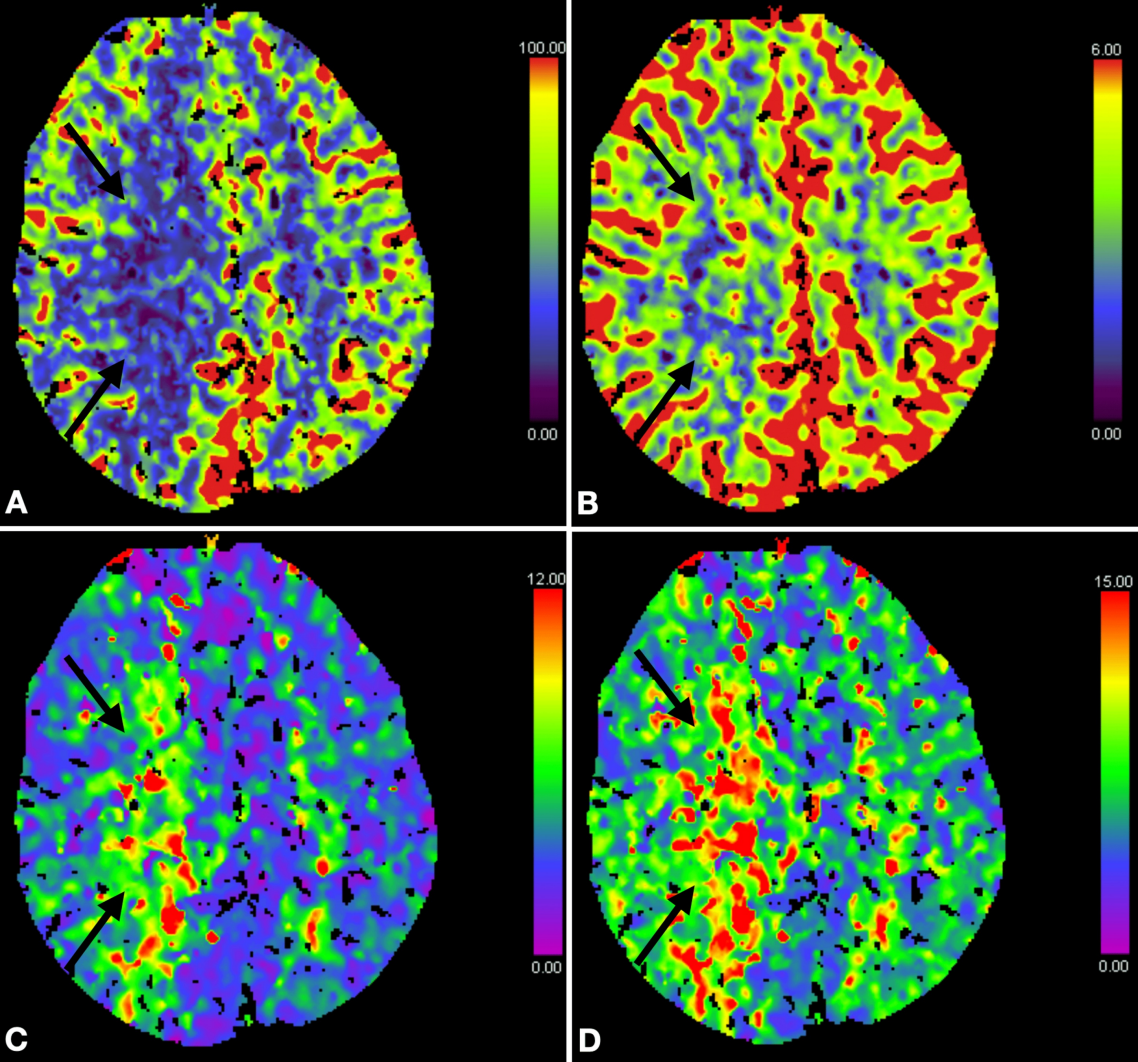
Computed tomography perfusion maps (A) Cerebral blood flow, (B) Cerebral blood volume, (C) Time to maximal perfusion, and (D) Time to peak perfusion. A perfusion deficit is evident in the posterior and internal watershed zones on the right (arrows).
